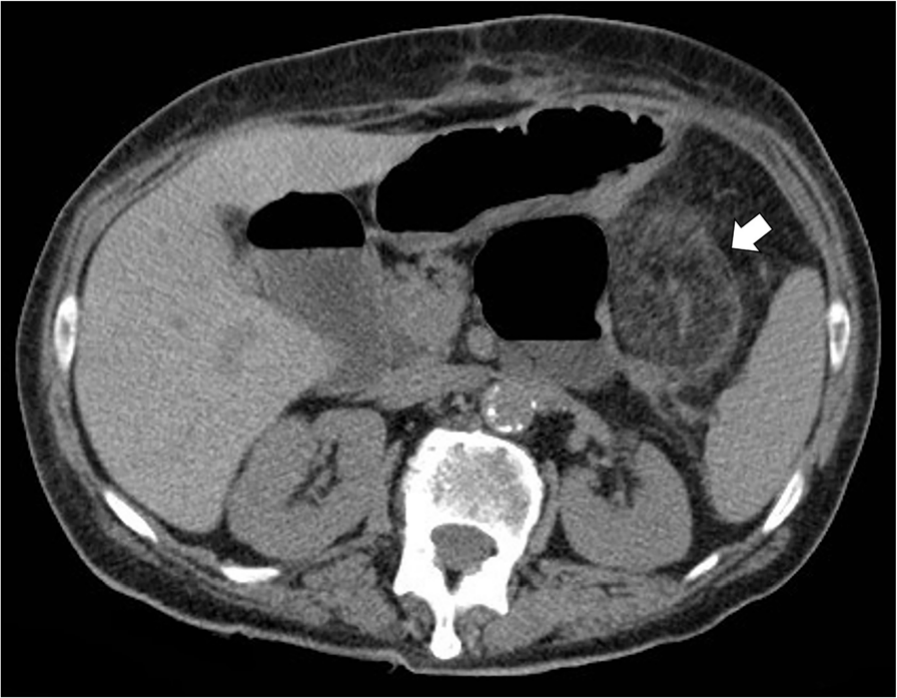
Acute omental infarction in a 67-year-old woman with previous history of total colectomy. Axial non-contrast CT image shows a 6.0 cm heterogeneous fatty ovoid lesion in the left upper quadrant (arrow), associated with inflammatory changes in the nearby fatty tissue
